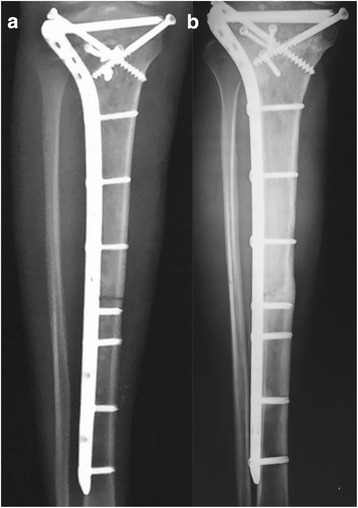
a Anteroposterior radiographs of right tibia after osteosarcoma resection and intercalary allograft reconstruction. b Anteroposterior radiographs of right tibia 21 months after the initial surgery
